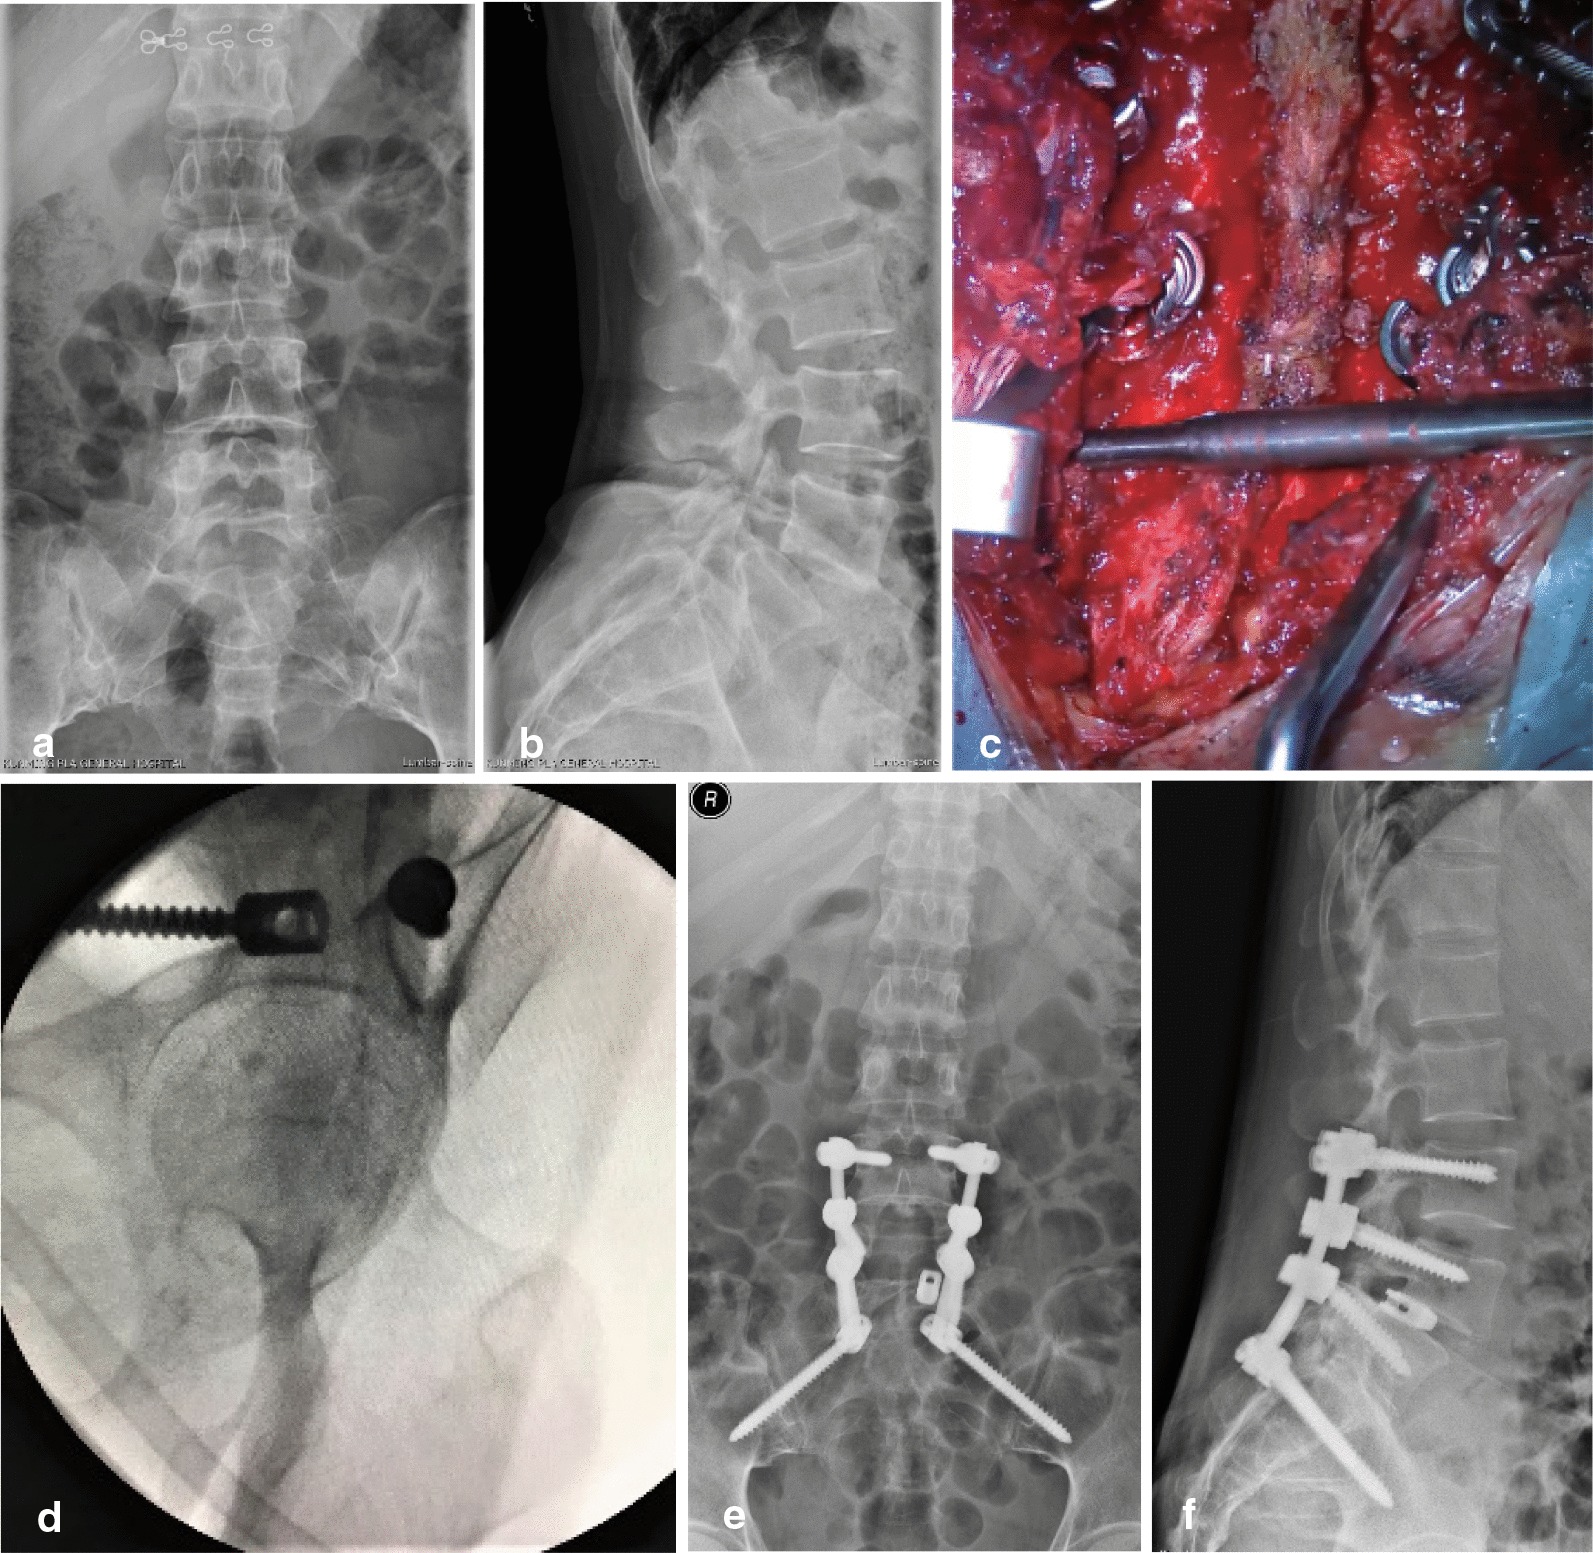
A 38-year-old women with lumbar spondylolisthesis (L5) and unstable spine. Anteroposterior-view radiograph (a) and lateral-view radiograph (b) before surgery. After selecting the screw entry point, a pedicle drill was projected to the site from the lower edge of the first superior articular process of the first sacrum to the first posterior sacral foramina on the contralateral side of the entry point, and the pedicle drill was close to the upper edge of the supraspinous ligament (c). After completion of screw placement, the radiographic teardrop view (axial view of the screw) shows the position of the screw was satisfactory (d). Postoperative follow-up anteroposterior (e) and lateral views (f) show that the slipped vertebrae were reduced, and the S2AI screw and other screws were in the desired places
